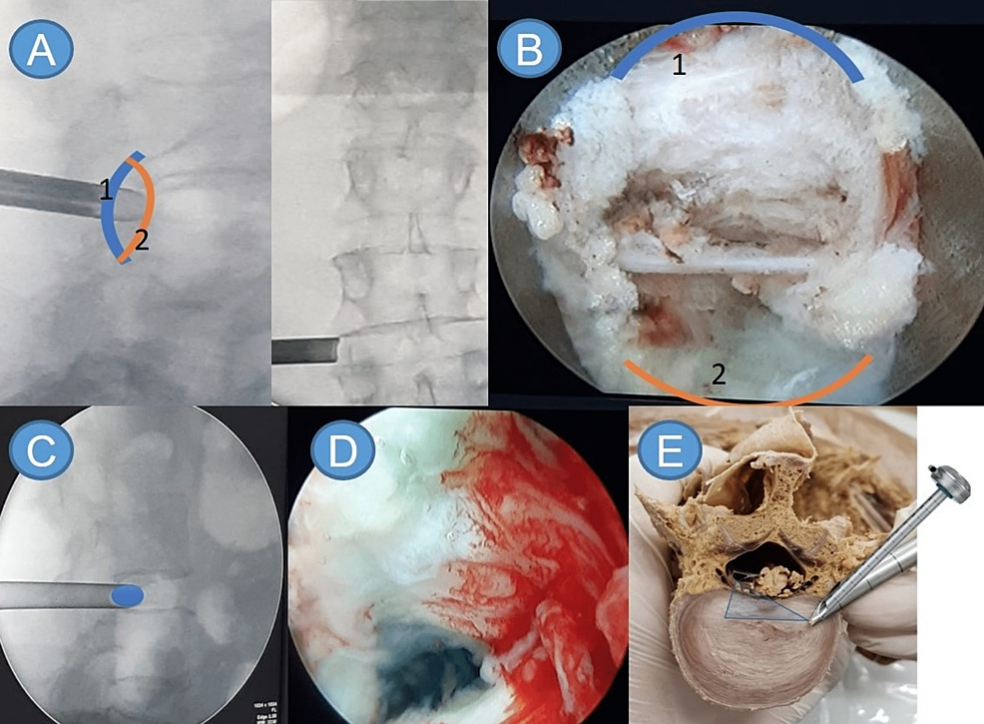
Radiological anatomy of the working sheath and endoscope facing the midline Configuration of the endoscopic view after retreating the endoscope a few millimeters from ‘’half and half". (A) Intraoperative imaging by profile radiography of the lumbosacral spine; (B) endoscopic view. A: bevel base; B: bevel tip. On the lateral radiography, the bevel base (A) is on the facet line, so when we look at the video screen, everything adjacent to it will be the facet. The bevel tip (B) is touching the disc, so on the video screen, the disc will be seen adjacent to it. (C) Intraoperative imaging confirming the endoscope’s position (profile lumbar spine radiography); (D) endoscopic view of the working area; (E) anatomical model representation (L2) of the correct position of the endoscope and the reference triangle that will appear on the video feed. Inside-out approach: the base and the tip are inside the disc and when entered with the endoscope, only the disc is observed. Source: author
